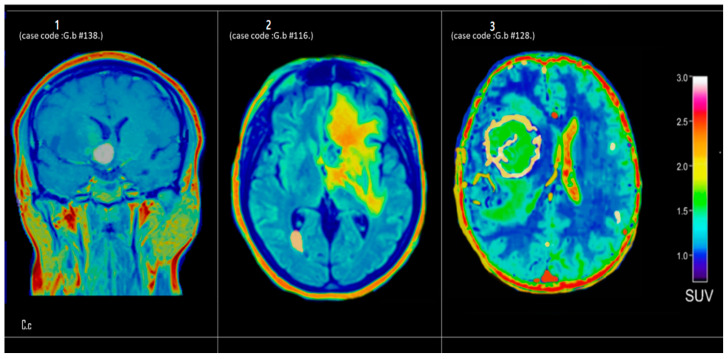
Axial ^18^F-FDG PET brain scans from Center C demonstrating distinct metabolic profiles. Internal case codes (G.b-#138, G.b-#116, G.b-#128) maintain data traceability. Panel 1 (G.b-#138): mild to moderate midline uptake (SUV 2.0–2.4), potentially indicating physiological variation or inflammatory activity. Panel 2 (G.b-#116): extensive periventricular hypermetabolism with focal hotspots (SUV ~3.0), suggestive of high-grade glioma or aggressive inflammatory condition. Panel 3 (G.b-#128): ring-like configuration of elevated uptake (SUV 2.8–3.2) surrounding hypometabolic center, characteristic of high-grade tumor or cerebral abscess.
